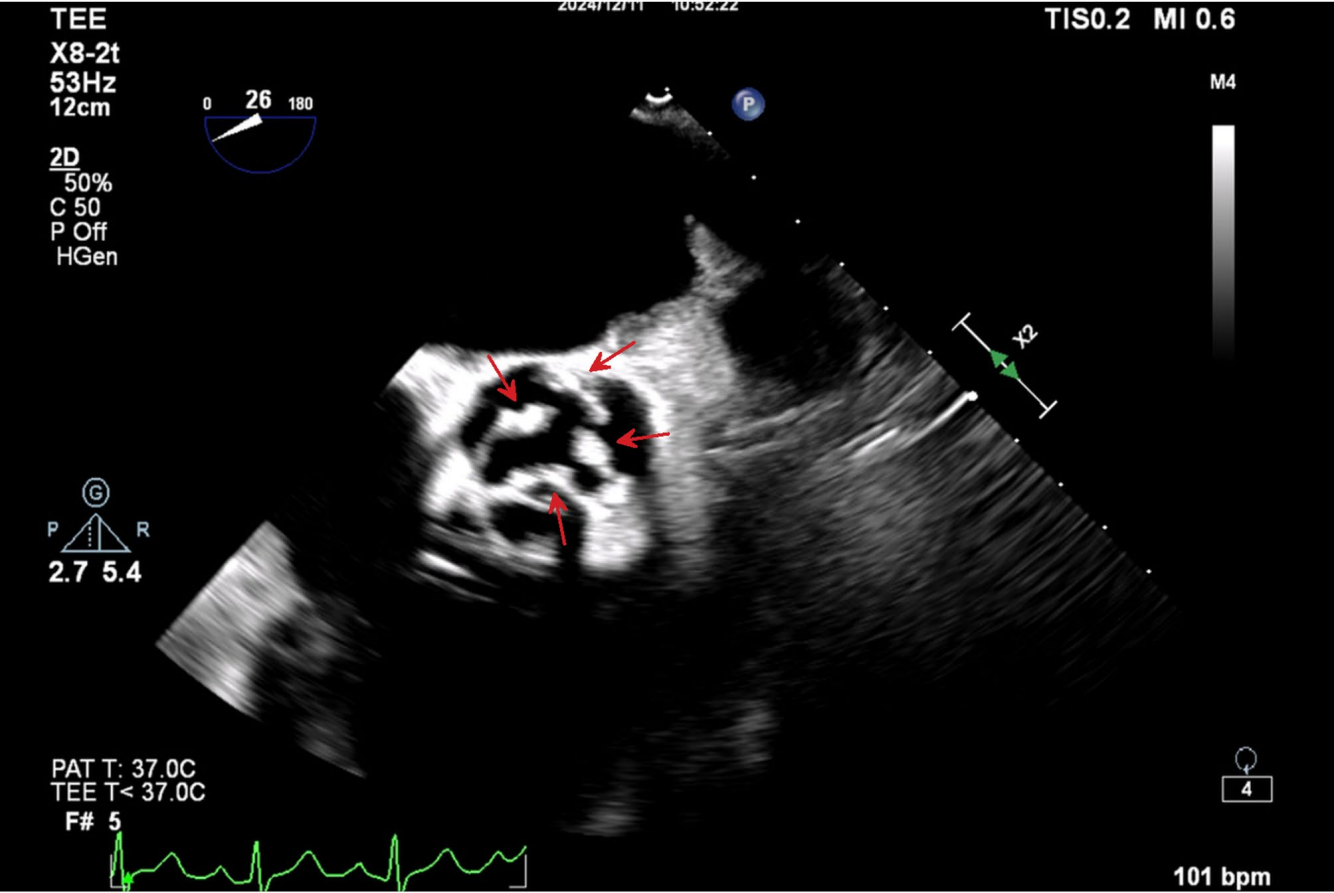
Transesophageal echocardiogram (TEE) showing aortic valve vegetation. TEE reveals multiple irregular, high-density vegetations attached to the aortic valve and annular root (red arrows), with measured sizes of 0.29 × 0.26 cm, 0.51 × 0.35 cm, 0.81 × 0.25 cm, and 0.54 × 0.32 cm
